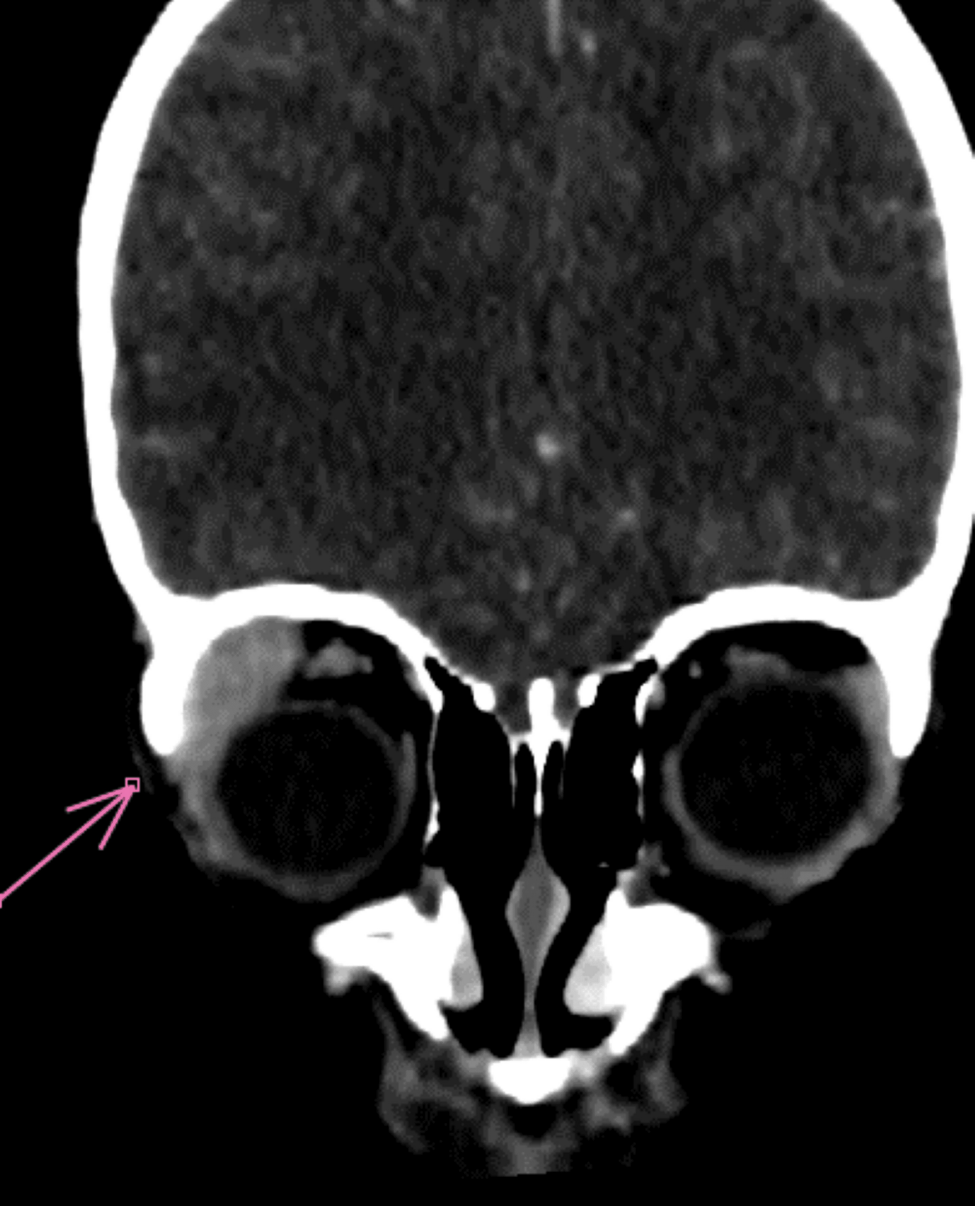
Coronal non-contrast brain CT scan showing infiltration of the lacrimal gland with extraconal extension
